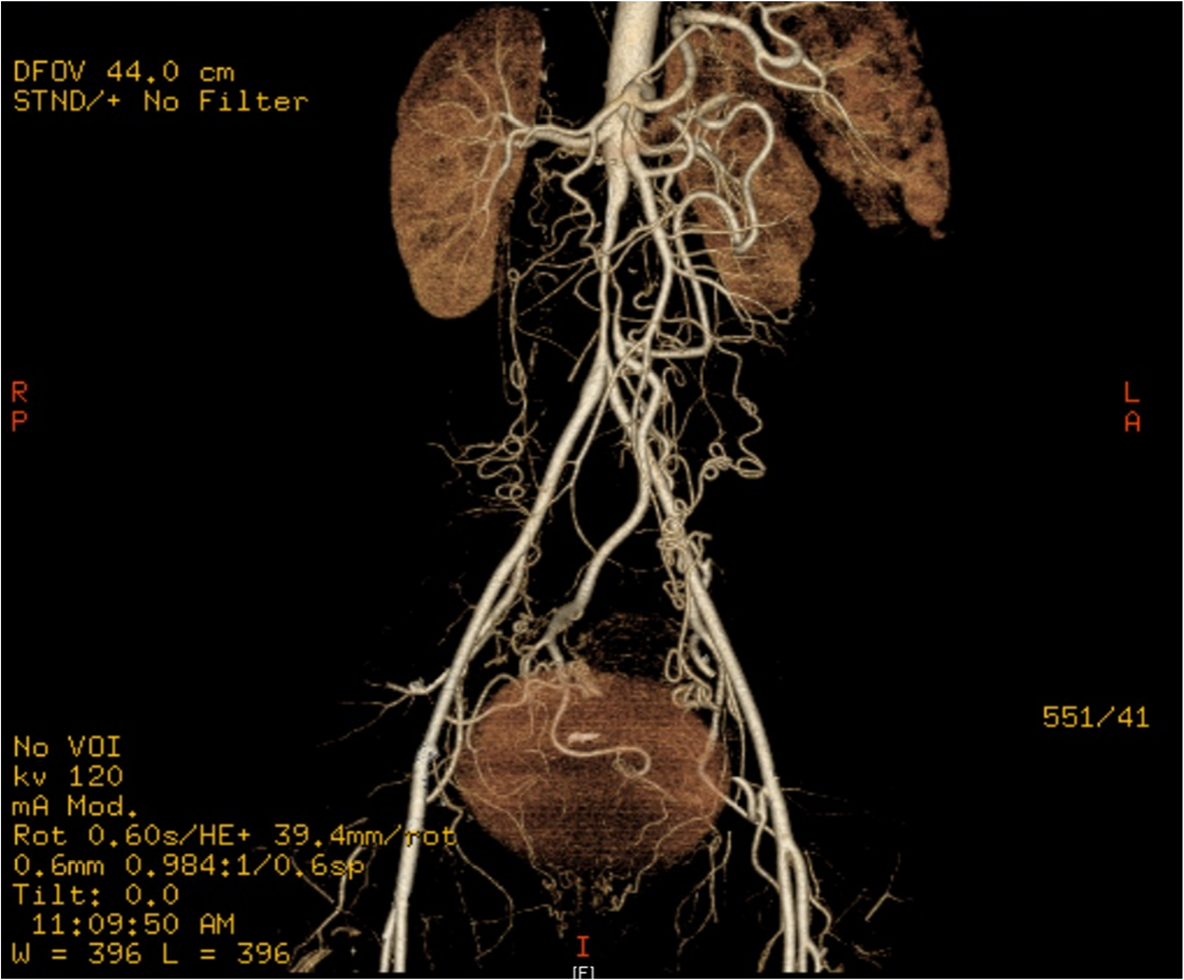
CT angiogram showing 75% infrarenal aortic stenosis with collateral circulation from the internal mammary and epigastric arteries
